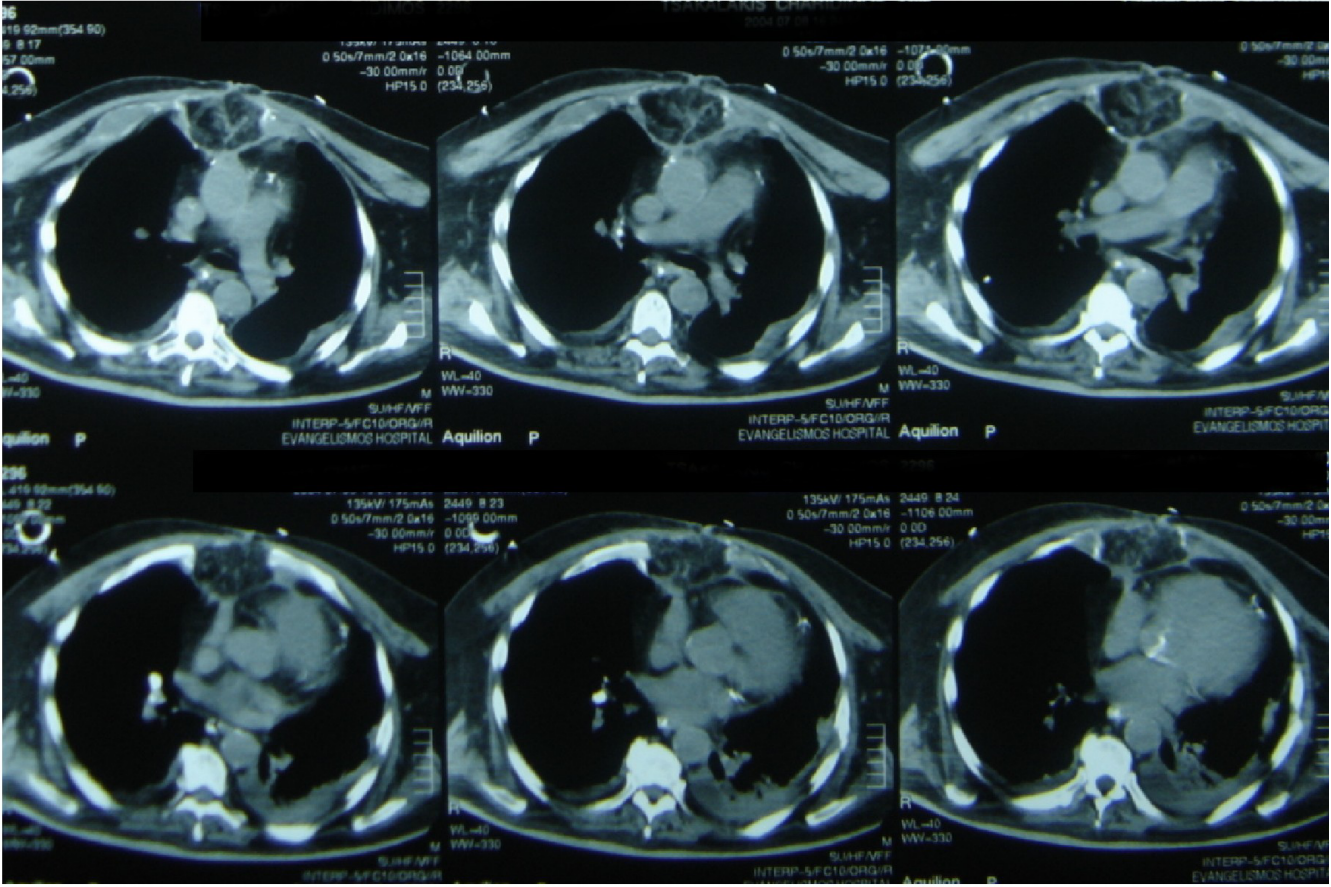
Result of the omental transfer one year after the operation in a chest CT scan.
